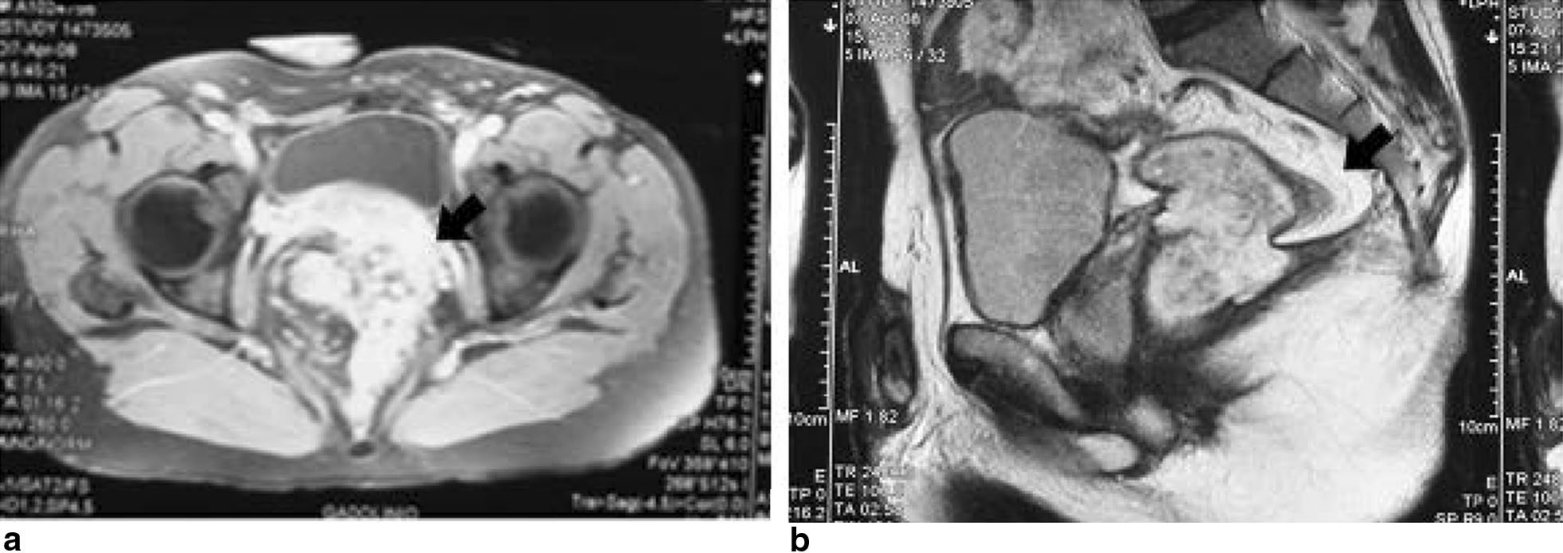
a MRI transverse plane b sagittal plane. An inhomogeneous pelvic mass of 7 × 7 × 4 cm in size, with thick and vascularised walls (*arrow*) is visible, compressing the bladder anteriorly, reaching the ano-rectal junction inferiorly and communicating with rectal lumen
